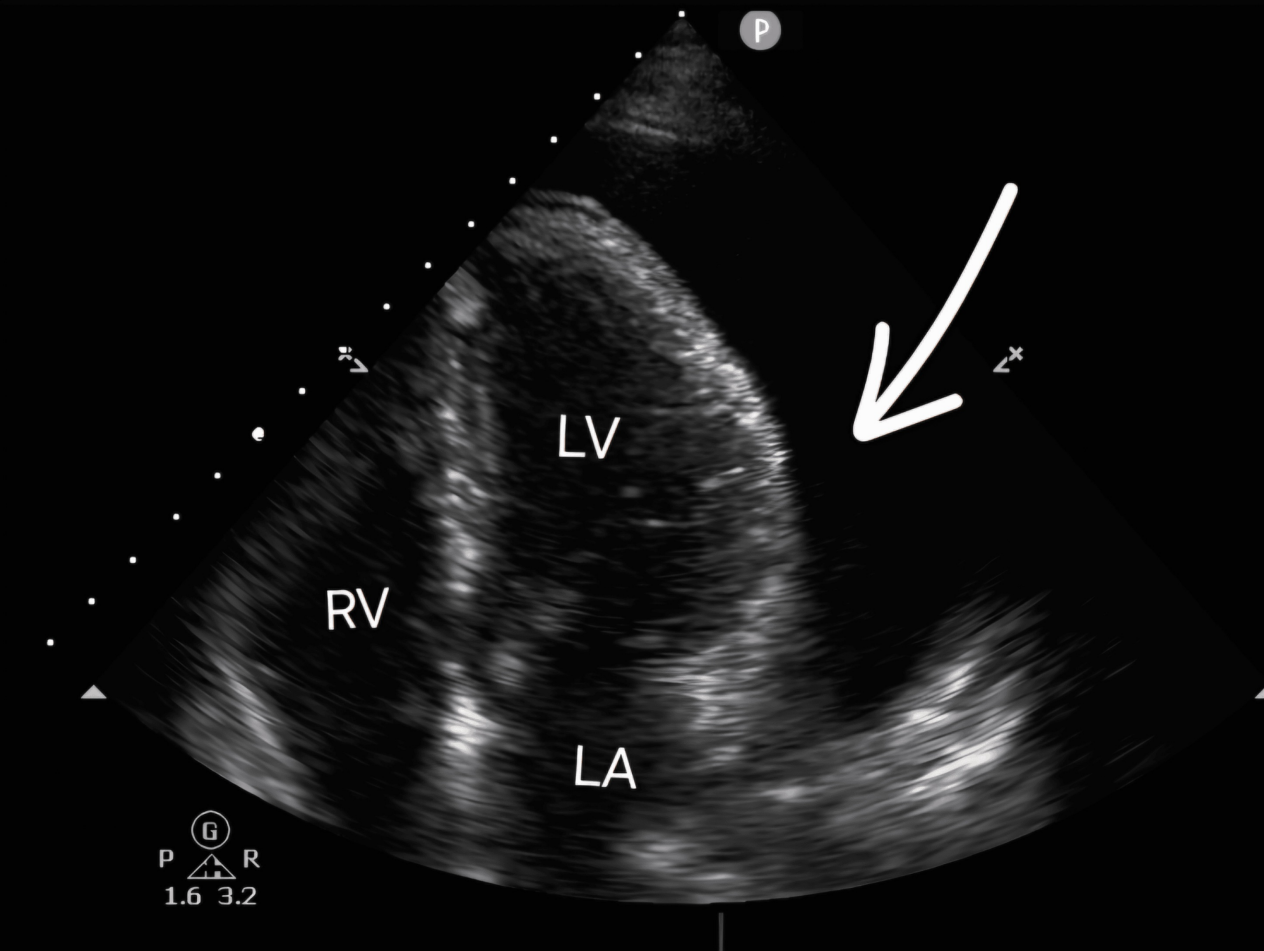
Apical four-chamber transthoracic echocardiogram showing a large circumferential pericardial effusion (white arrow), visible as an anechoic space surrounding the heart. The RV appears compressed, consistent with diastolic collapse, a hallmark of cardiac tamponade. The LV and LA remain structurally preserved RV: right ventricle; LV: left ventricle; LA: left atrium
